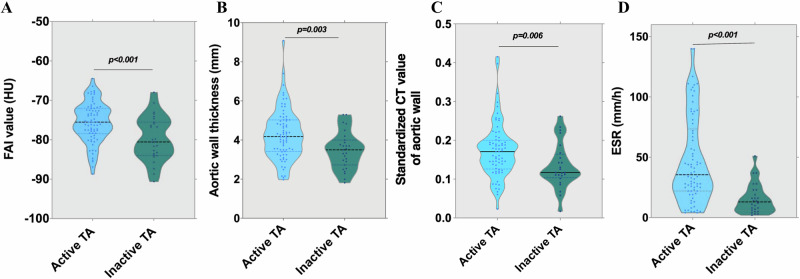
Distribution of clinical and imaging markers by disease activity. A Violin plot showing the distribution of the FAI value. B Aortic wall thickness. C Standardized CT value of aortic wall. D ESR according to the activity status of TA. HU, Hounsfield unit; FAI, fat attenuation index; ESR, erythrocyte sedimentation rate; TA, Takayasu arteritis
